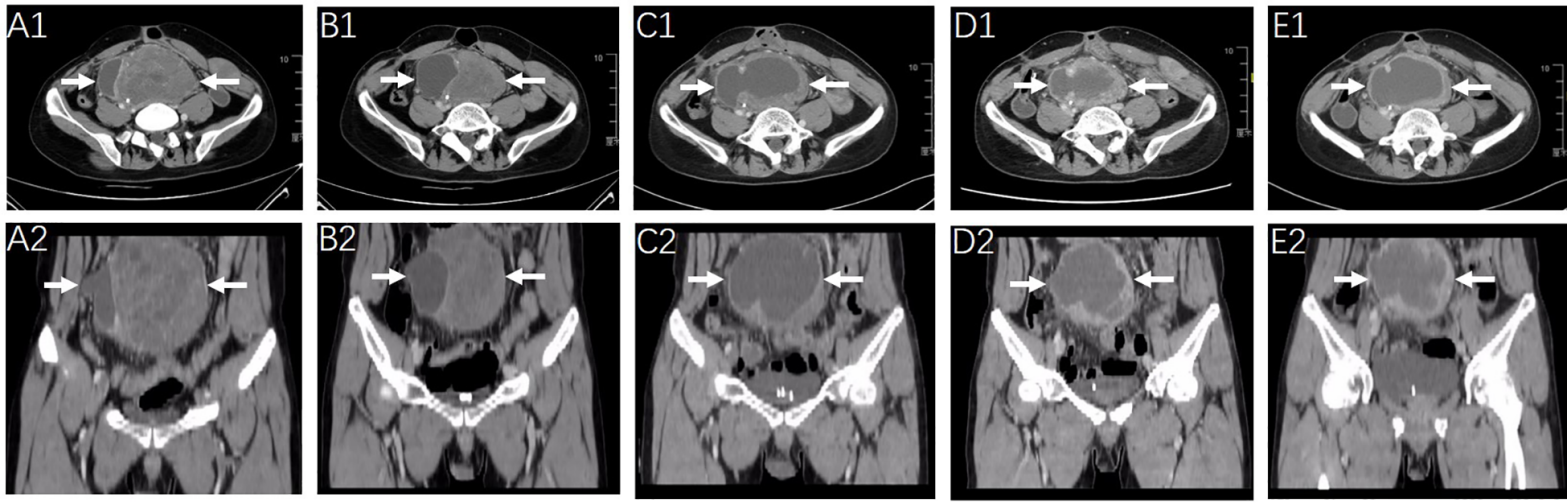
Radiologic response to the first-line treatment of radiotherapy combined with anlotinib and sintilimab monoclonal antibody and the second line treatment of chemotherapy. CT scanning images of the abdominopelvic tumor mass (arrow) were obtained before the initiation of first-line on March 14, 2022 (A1, A2) and after 2-months of therapy on May 12, 2022 (B1, B2). Representative images of the abdominopelvic tumor mass (arrow) on July 2022 [before chemotherapy, (C1, C2)] September 2022 [after 3 cycles of chemotherapy, (D1, D2)] and December 2022 [after 6 cycles of chemotherapy, (E1, E2)].
